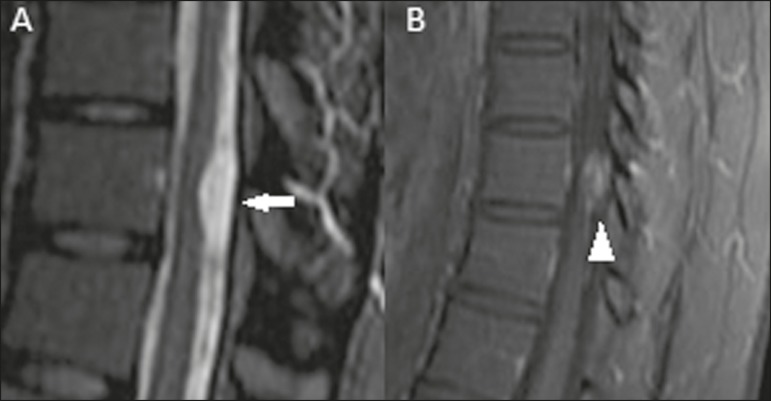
Sagittal T2-weighted MRI sequence (A) and contrast-enhanced
